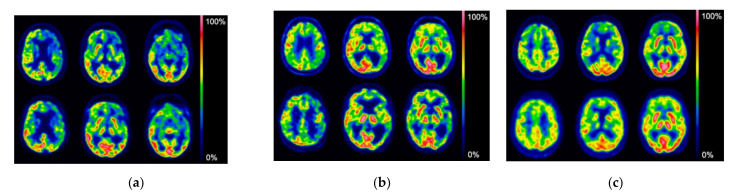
Three representative axial brain slices of [^18^F]FDG PET images (upper row) and their corresponding FMF for each Aβ radiotracer (lower row) are shown using a rainbow color scale in the Xeleris processing system (GE Healthcare, Chicago, IL, USA). (a) [^18^F]FBB FMF: 68-year-old male with mild dementia and non-fluent variant PPA (nfvPPA), suggests FTD. [^18^F]FDG PET and [^18^F]FBP FMF show severe left frontal involvement and left parietal and temporal involvement. The amyloid PET scan was negative. (b) [^18^F]FMM FMF: 73-year-old male diagnosed with possible AD and amnestic long-term cognitive decline, and as an amnesic, with an MRI with atrophy, and a history of alcoholism. [^18^F]FDG PET and [^18^F]FMM FMF show severe left parietal and frontotemporal involvement. The amyloid PET scan was positive. (c) [^18^F]FBP FMF: 69-year-old woman diagnosed with probable AD due to hippocampal amnestic deterioration. Both the [^18^F]FDG PET and [^18^F]FBB FMF images show slight bilateral parietal involvement which is greater on the right side and slight right frontal involvement. The amyloid PET scan was positive.
